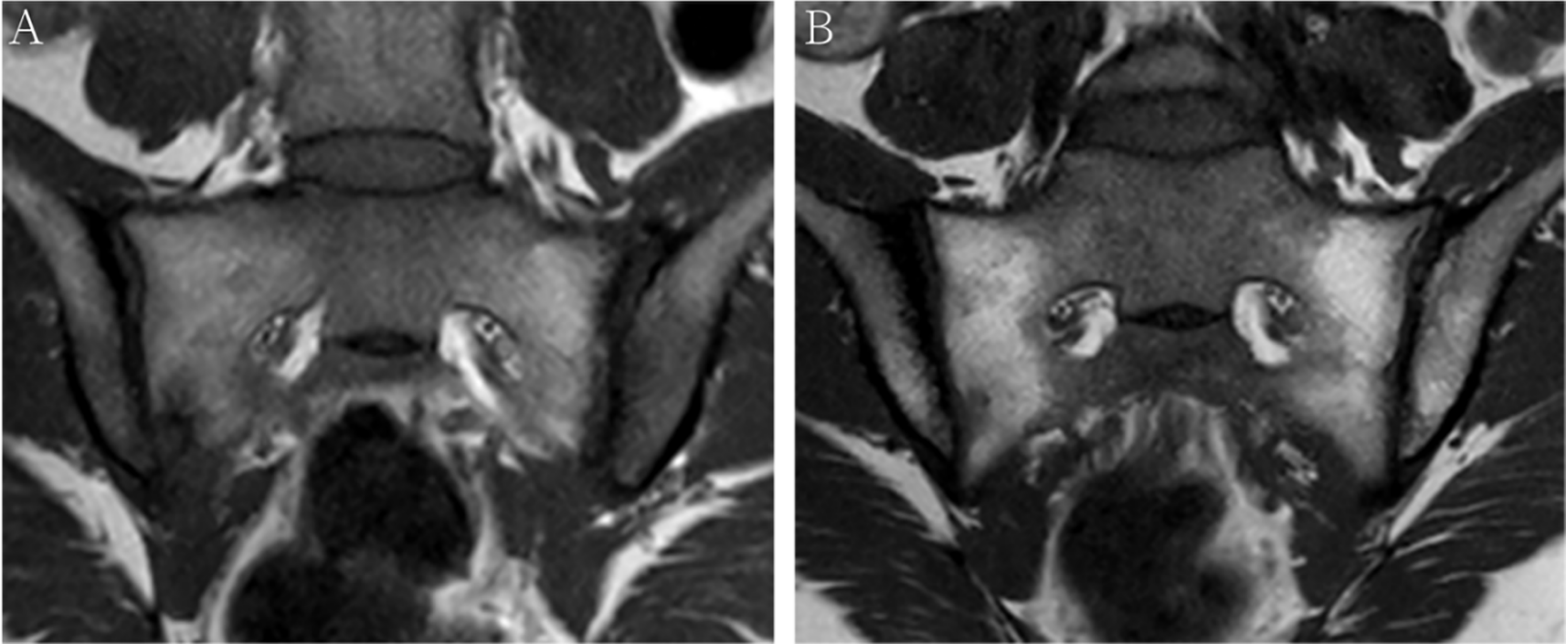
MRI findings of a 38-year-old female with HLA-B27 negative axial spondyloarthritis at baseline (A) and 12-month follow-up (B). Coronal oblique T1 images show fat metaplasia at both sacroiliac joint surfaces, however, no backfill occurs after 12-month follow-up.
